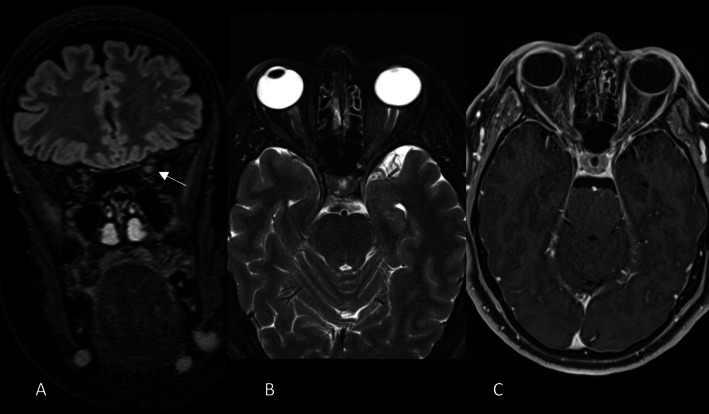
MR shows subtle hyperintensity along the optic nerve in FLAIR coronal images (arrow in A). No significant swelling of the nerves in T2 axial fat sat images (B) nor clear enhancement in T1 after gadolinium administration (C)
